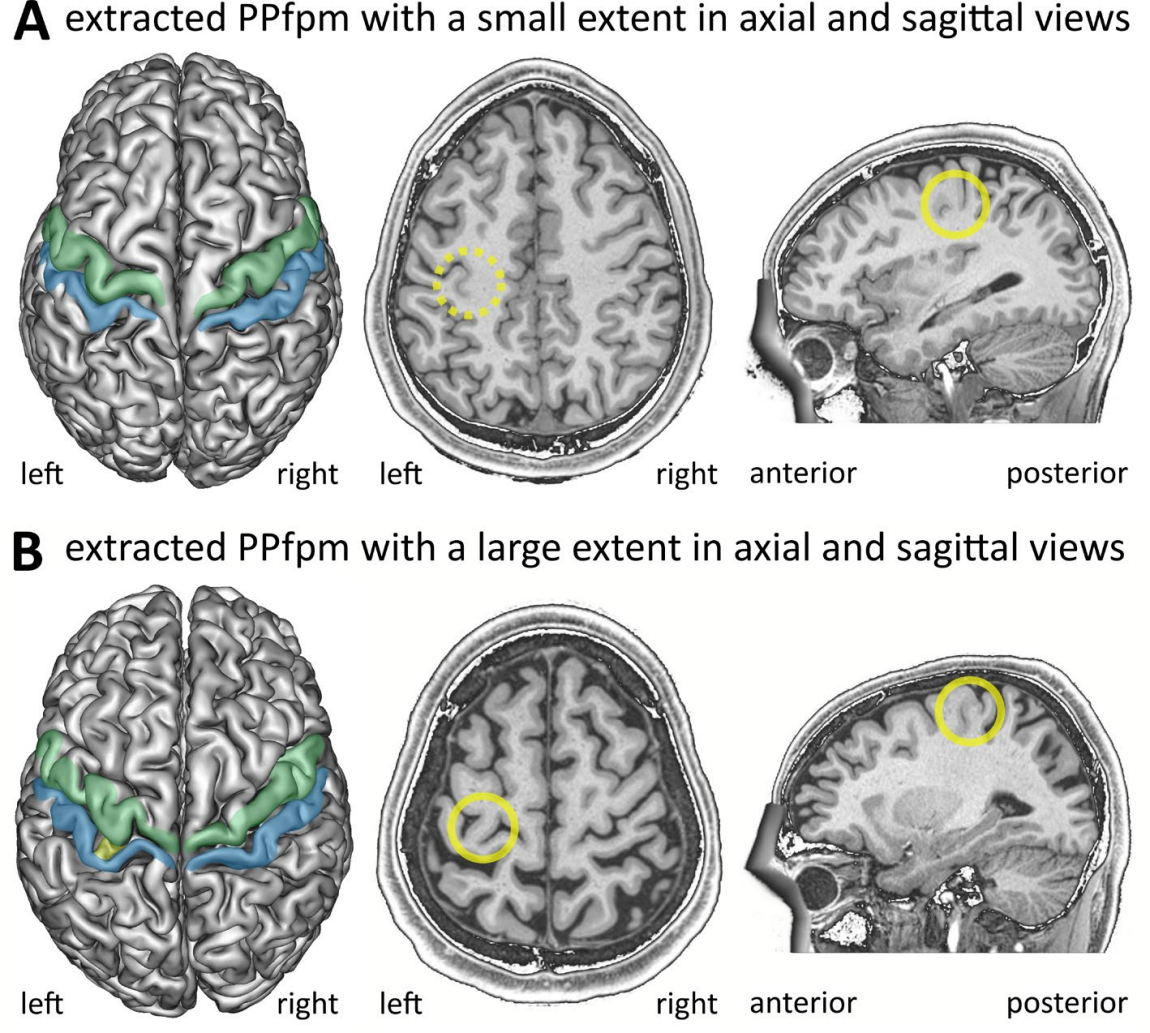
The PPfpm as seen on the cortical surface and in standard MRI views in native space. For two exemplary subjects of the HCP‐YA, cortical grey matter reconstructions are shown in relation to the anatomy as seen in standard axial and sagittal MRI. Subjects display a PPfpm of (A) a small and (B) a large extent in the left hemisphere. Left: Cortical grey matter reconstructions with marked precentral gyrus (green), postcentral gyrus (blue) and PPfpm (yellow). Note that the PPfpm is not visible in cortical grey matter reconstructions when its extent is small but is apparent when its extent is large (B). Middle: Axial MRI view with the PPfpm marked with a yellow circle. Note that a PPfpm with a small extent (A) is not clearly demarked in axial views; the dotted circle indicates the position of the PPfpm. Only a PPfpm with a large extent (B) can be visualized as a distinct cerebral fold. Right: Sagittal MRI view with the PPfpm marked in a yellow circle. The PPfpm is generally detectable as a distinct connection between the precentral hook of the hand knob and a counter‐hook of the postcentral gyrus. Note that the PPfpm can be detected in sagittal views even if it is of only a small extent.
